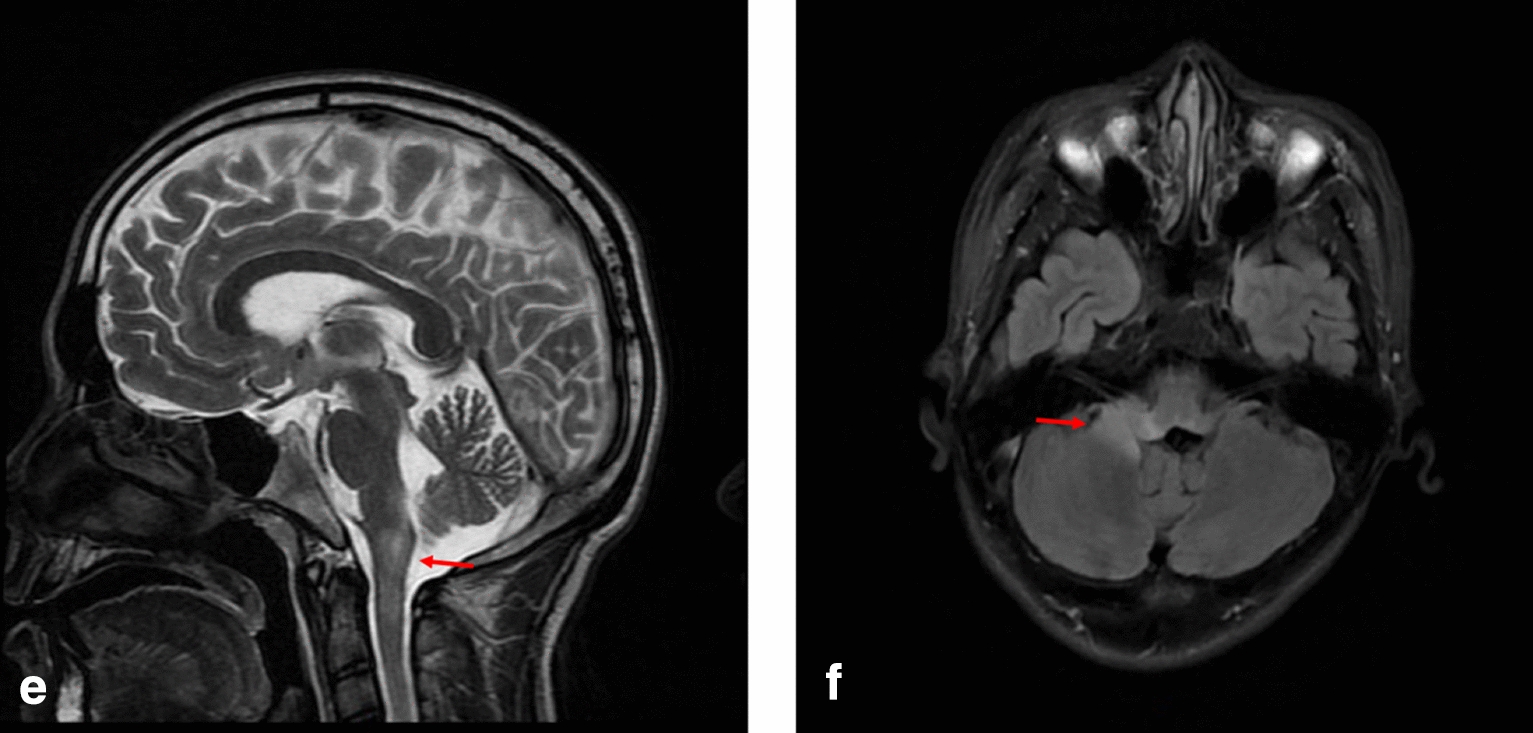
MRI T2 sequence of case 3 showing hyperintense in medulla oblongata (highlighted by arrows) in the e sagittal planes, and hyperintense in the right cerebellum and brainstem in the f transverse planes
